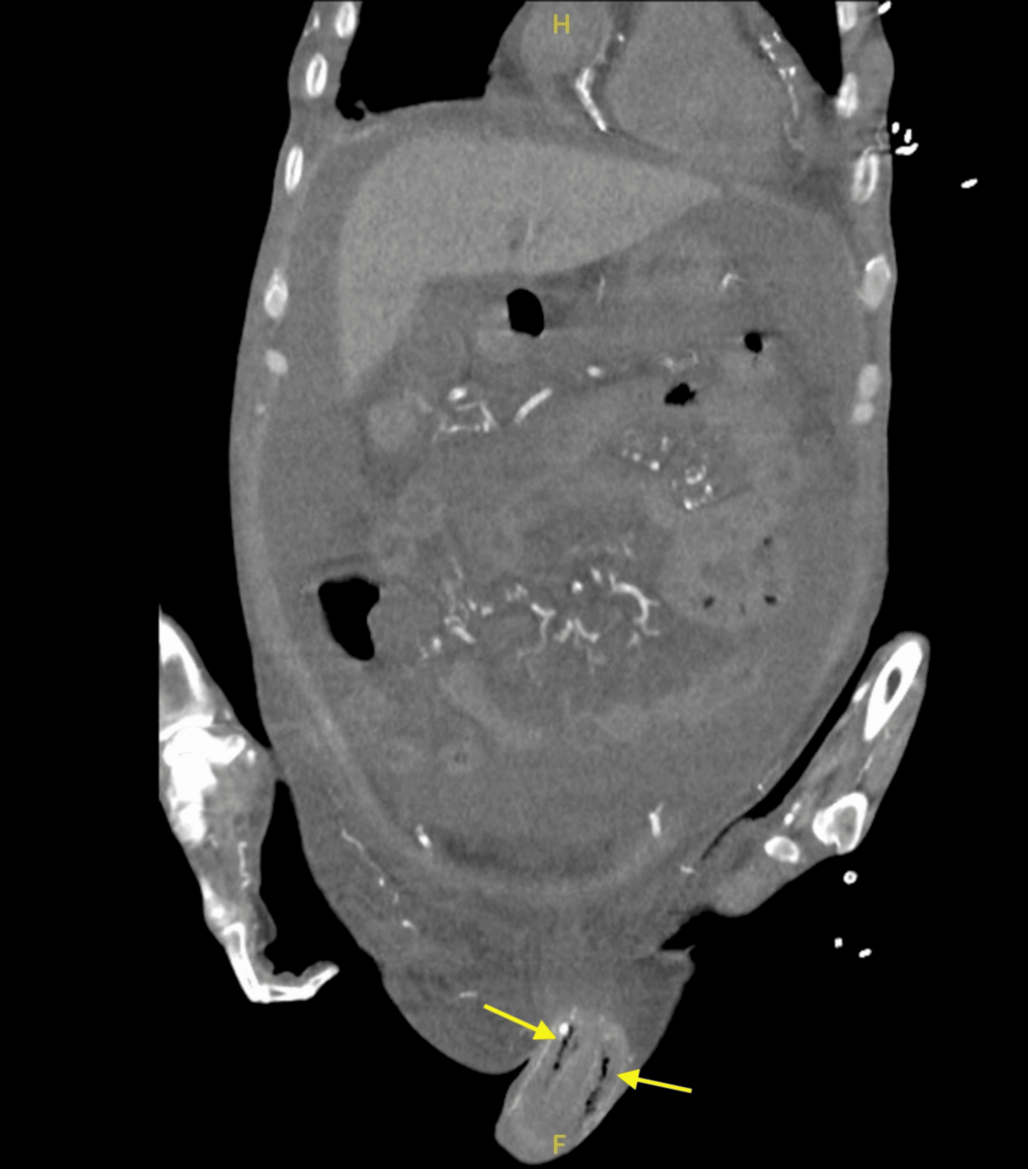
Coronal CT abdomen and pelvis without contrast showing subcutaneous gas within the corpus cavernosum of the penis extending to its base, with diffuse penile edema
